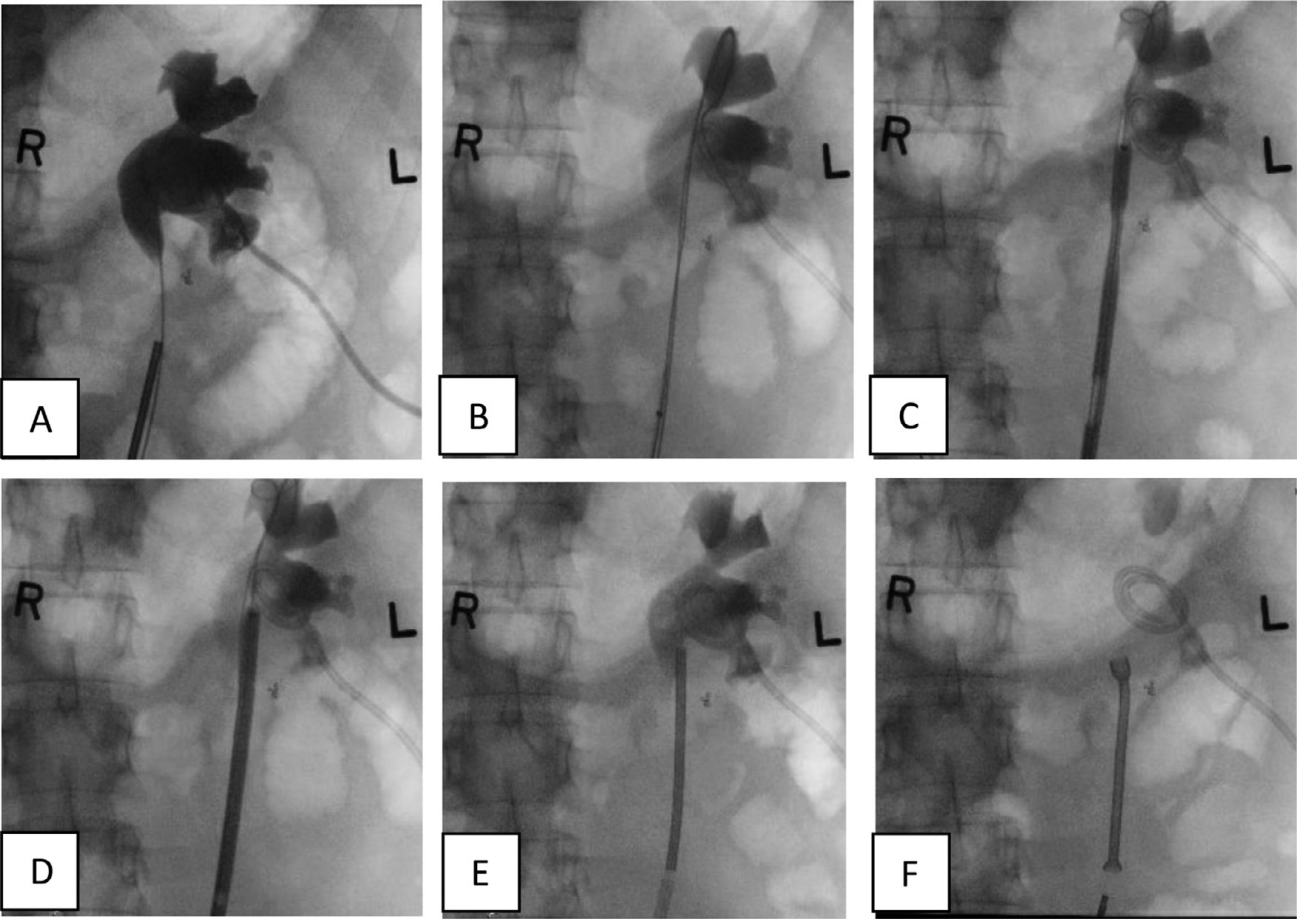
Intraoperative X-rays showing: Nephrostogram with upper ureteric blockage (A) bypass of the left upper ureteric stricture with guidewire and balloon dilatation (B-D). Memokath insertion in left upper ureter (E,F).
